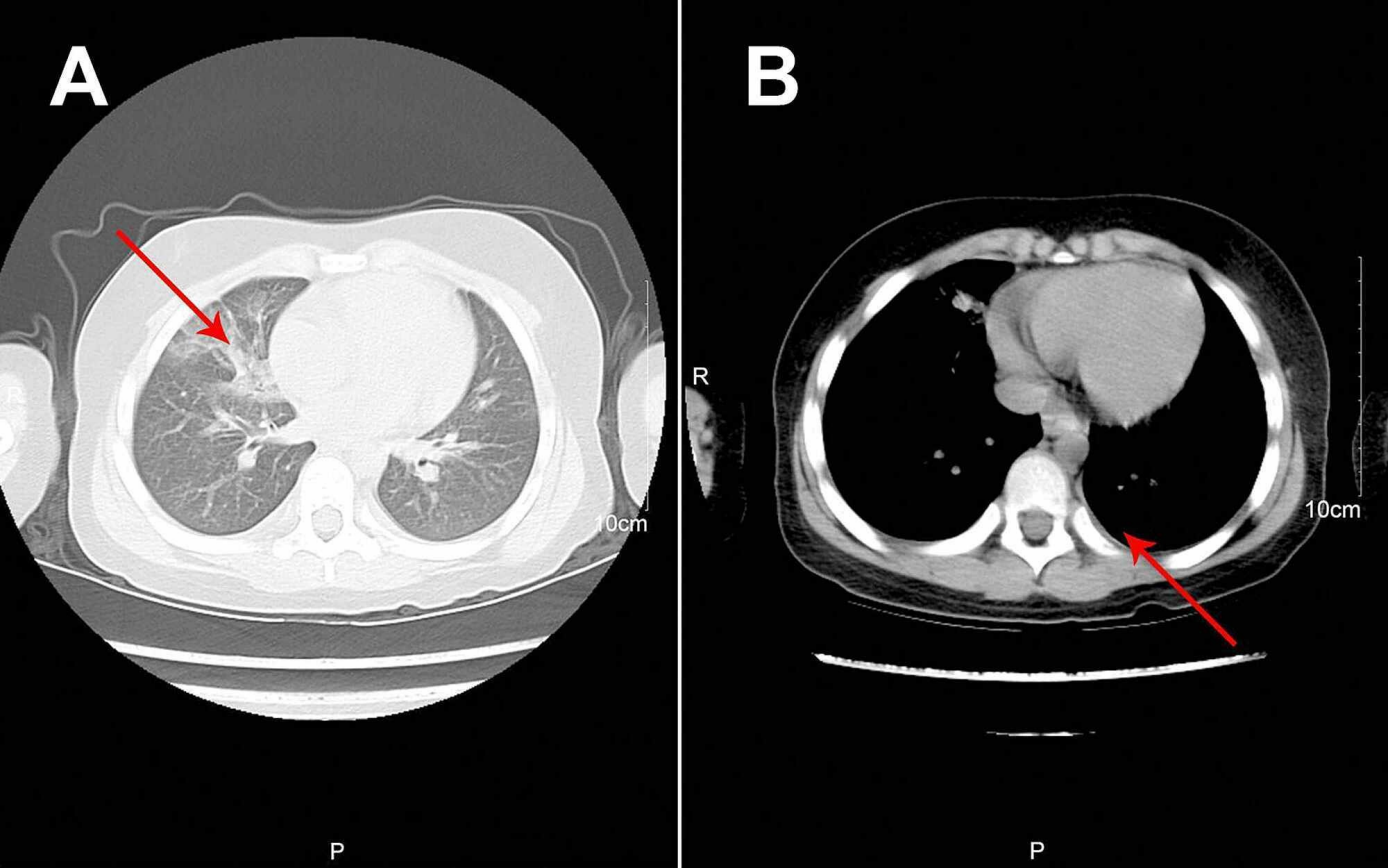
Reexamination of Chest Computerized Tomography (A) Chest CT reexamination after extubation showed full expansion of the left lung with pleural effusion on the left thorax and bilateral pneumonia and (B) chest CT for the first follow-up visit showed increased lung markings.
